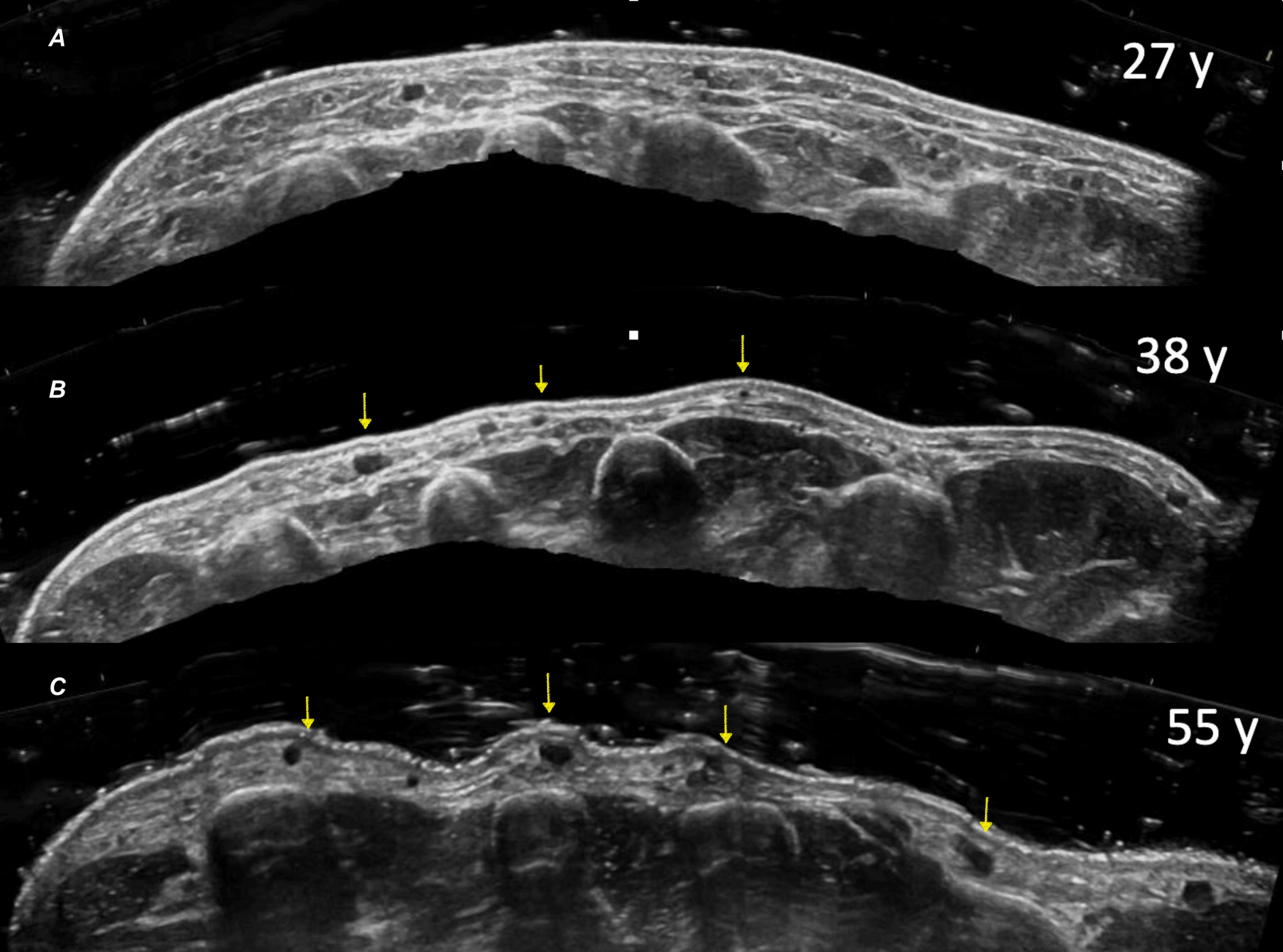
HRUD of dorsal hand aging patterns across the adult lifespan (transverse views) (3A) Woman aged 27 years with preserved subcutaneous fat and normal dorsal hand anatomy; (3B) Woman aged 38 years with moderate fat loss; yellow arrows highlight mild prominence of the dorsal venous plexus; (3C) Woman aged 55 years with marked fat loss; yellow arrows indicate pronounced venous plexus prominence. Abbreviations: HRUD, high-resolution ultrasound with Doppler.
